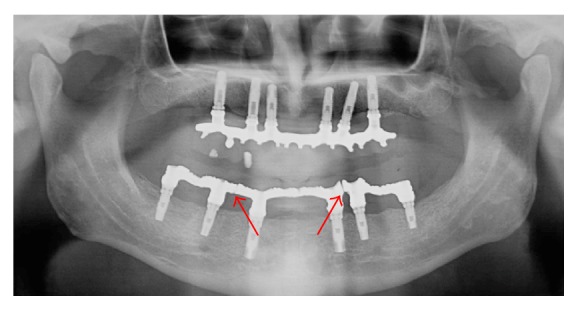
Panoramic radiograph after a year of installation of the mandibular fixed implant-supported prosthesis. Red arrows show the cuts in framework.
